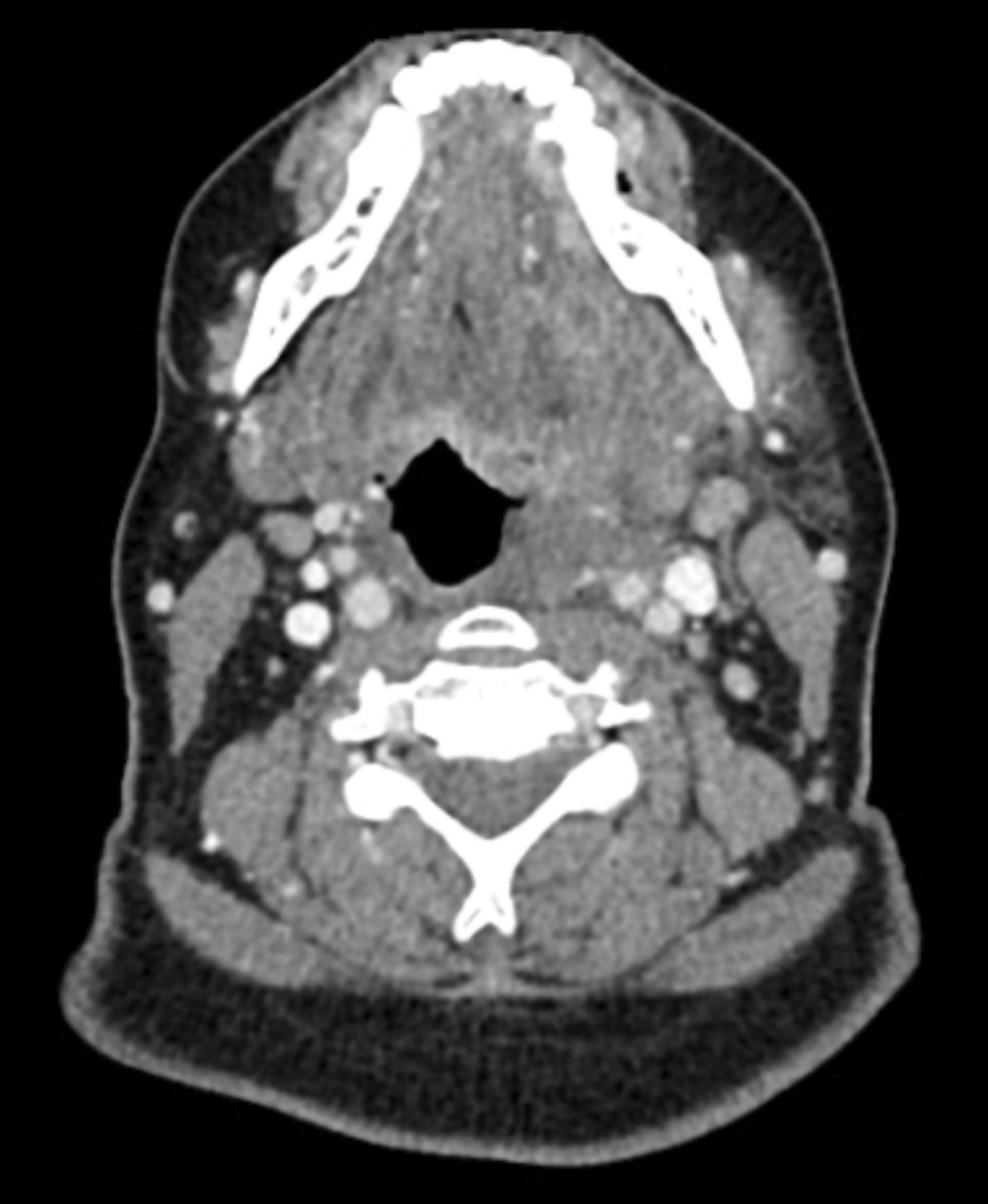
Contrast‐enhanced computed tomography (CT) imaging demonstrating a soft tissue abnormality within the left parapharyngeal space adjacent to the oropharynx but without convincing evidence of enhancement, suggestive of an inflammatory phlegmon.
